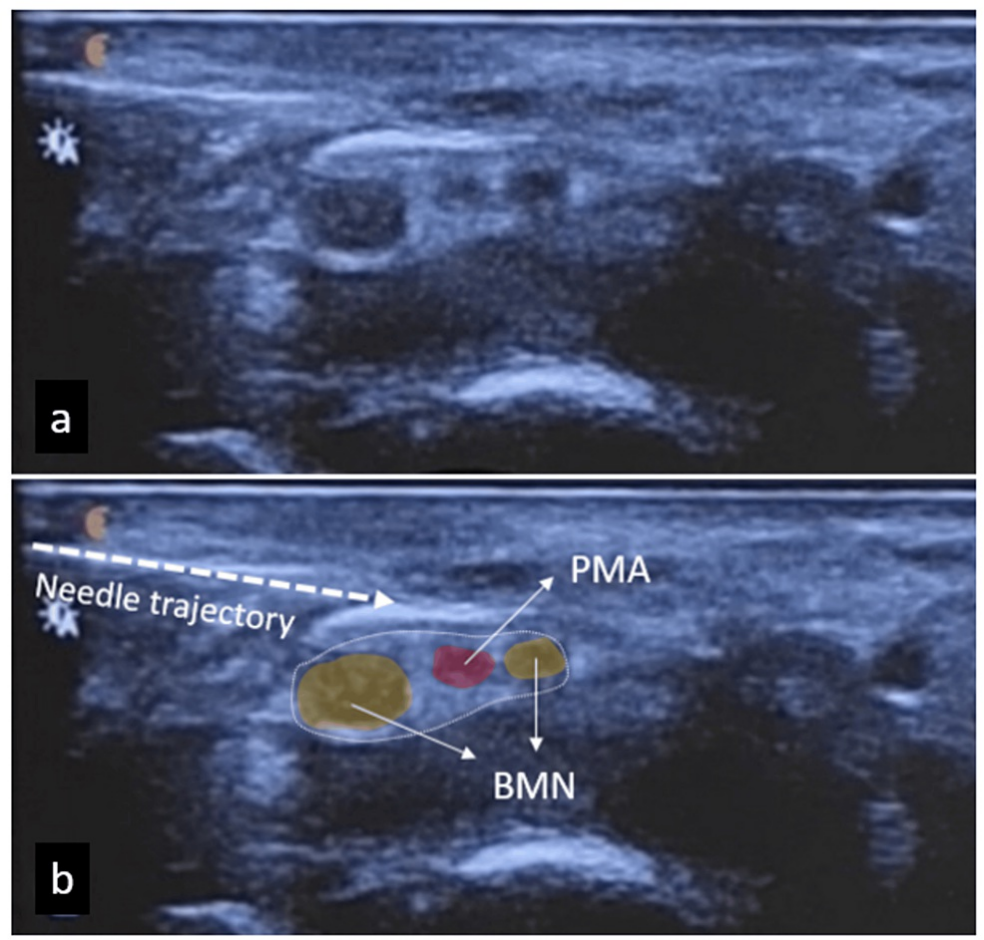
(a) Transverse ultrasonography of the right wrist showing the bifid median nerve and the persistent median artery. The image also shows ultrasound-guided injection and needle trajectory. The bottom image (b) is colored to distinguish the relevant structures PMA: persistent median artery (red), BMN: bifid median nerve (yellow), needle (dashed line)
